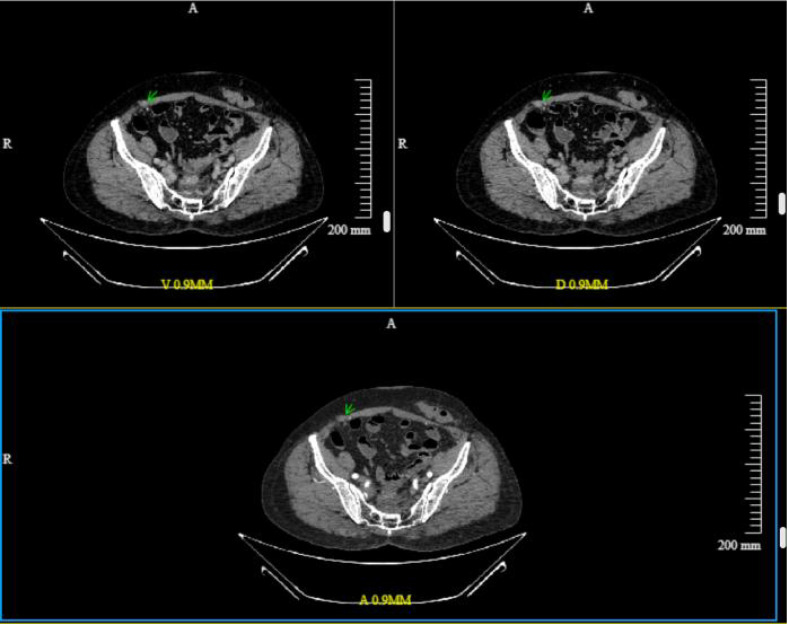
Contrast-enhanced CT scan in May 2024. The peritoneal nodule in the anterior abdominal wall (arrow) shows early enhancement in the arterial phase (lower middle) with visible intralesional vascularity, and persistent enhancement in the venous phase (upper left) and delayed phase (upper right), consistent with peritoneal metastasis.
